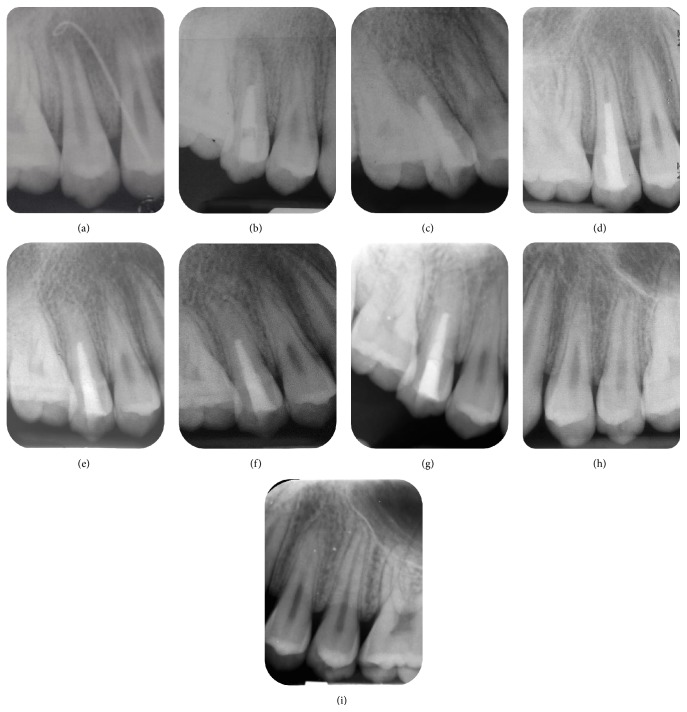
Periapical radiographs. (a) Preoperative, sinus tracing with gutta-percha. (b) After placement of MTA into the root canal. (c) 4-month follow-up showing healing of periapical lesion. (d) 7-month follow-up showing increase in root length. (e) 18-month follow-up showing apical closure. (f) 36-month follow-up showing a narrowed canal lumen. (g) 66-month follow-up showing absence of canal lumen. (h) Tooth 25 (control) at 7-month follow-up. (i) Tooth 25 (control) at 66-month follow-up.
